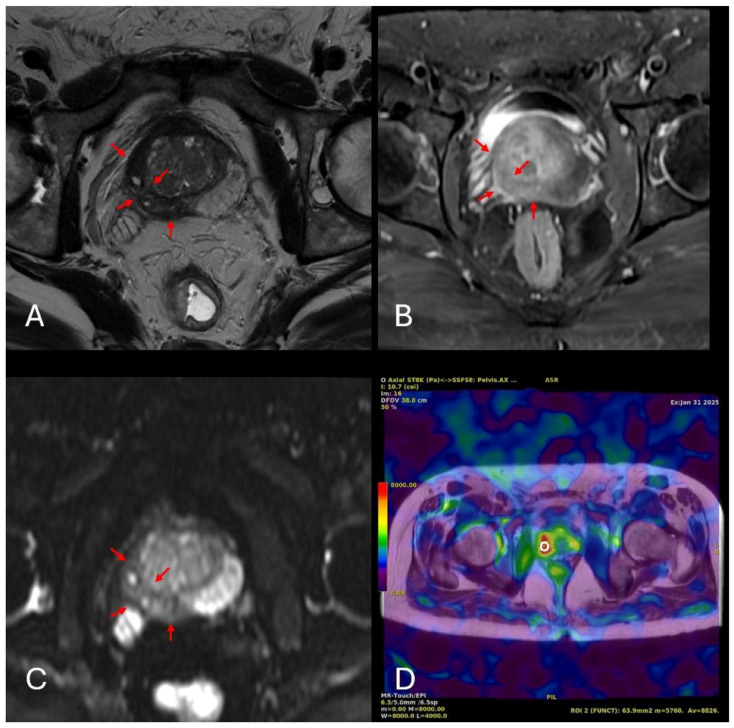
MRI and MRE findings of a 76-year-old patient presenting with dysuria, polyuria, and nocturia, with a PSA level of 5.8 ng/mL. Axial T2-weighted imaging (A) demonstrates marked T2 hypointensity in the right peripheral zone (red arrows). Post-contrast imaging (B) shows the corresponding contrast enhancement in the same region, whereas diffusion-weighted imaging (C) reveals significant diffusion restriction. In the corresponding MRE image (D), a region of interest (ROI; white open circle) was placed over a red-coded area representing the stiffest region within the parenchyma, yielding a stiffness value of 8.826 kPa.
